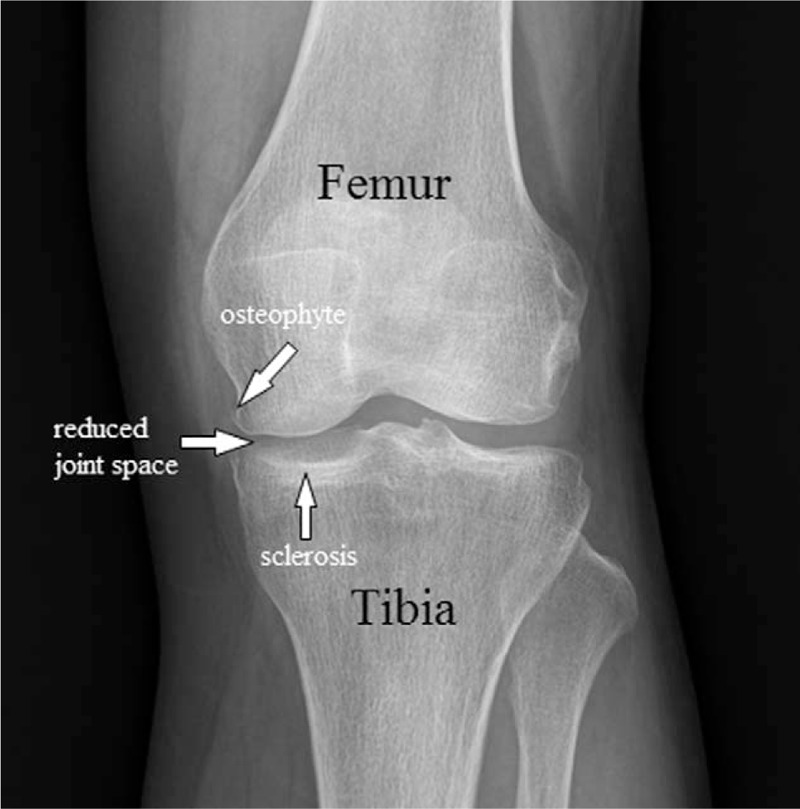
X-ray of the knee showing bone spurs, sclerosis, and a narrowed joint space caused by osteoarthritis.
